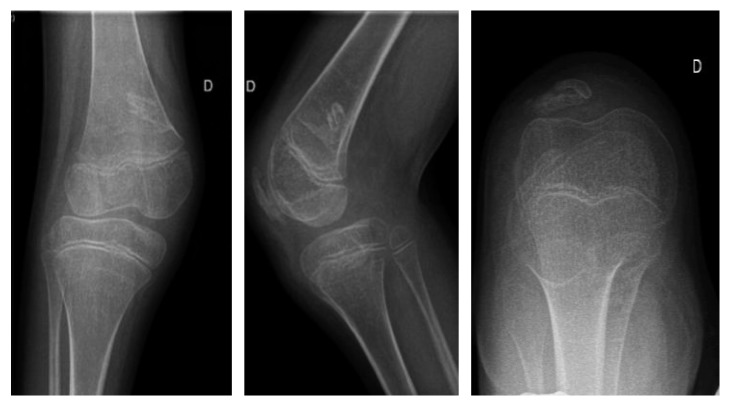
Patient 2: X-ray at follow-up. The patella is centered.
